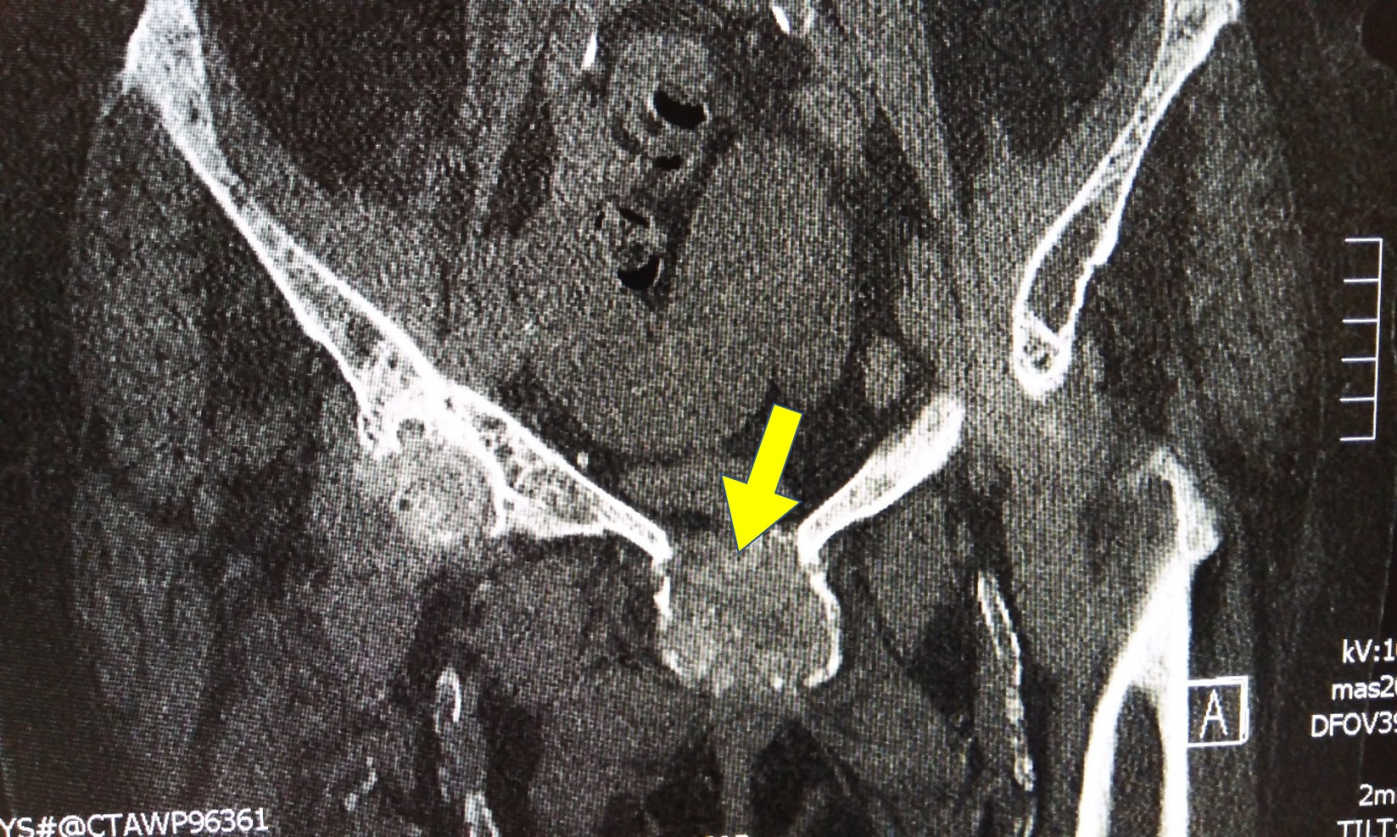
Computed tomography (CT) scan of the pelvis - axial view Chronic pubic symphysitis in the axial view.
